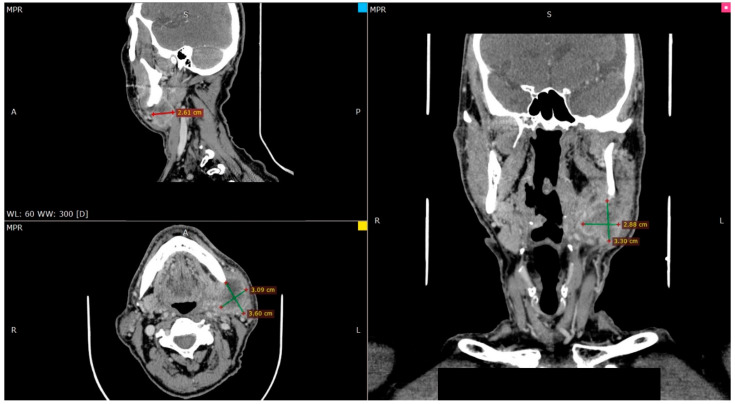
CT imaging of the initial neck mass. A: anterior, P: posterior, R: right, L: left, S: superior.
